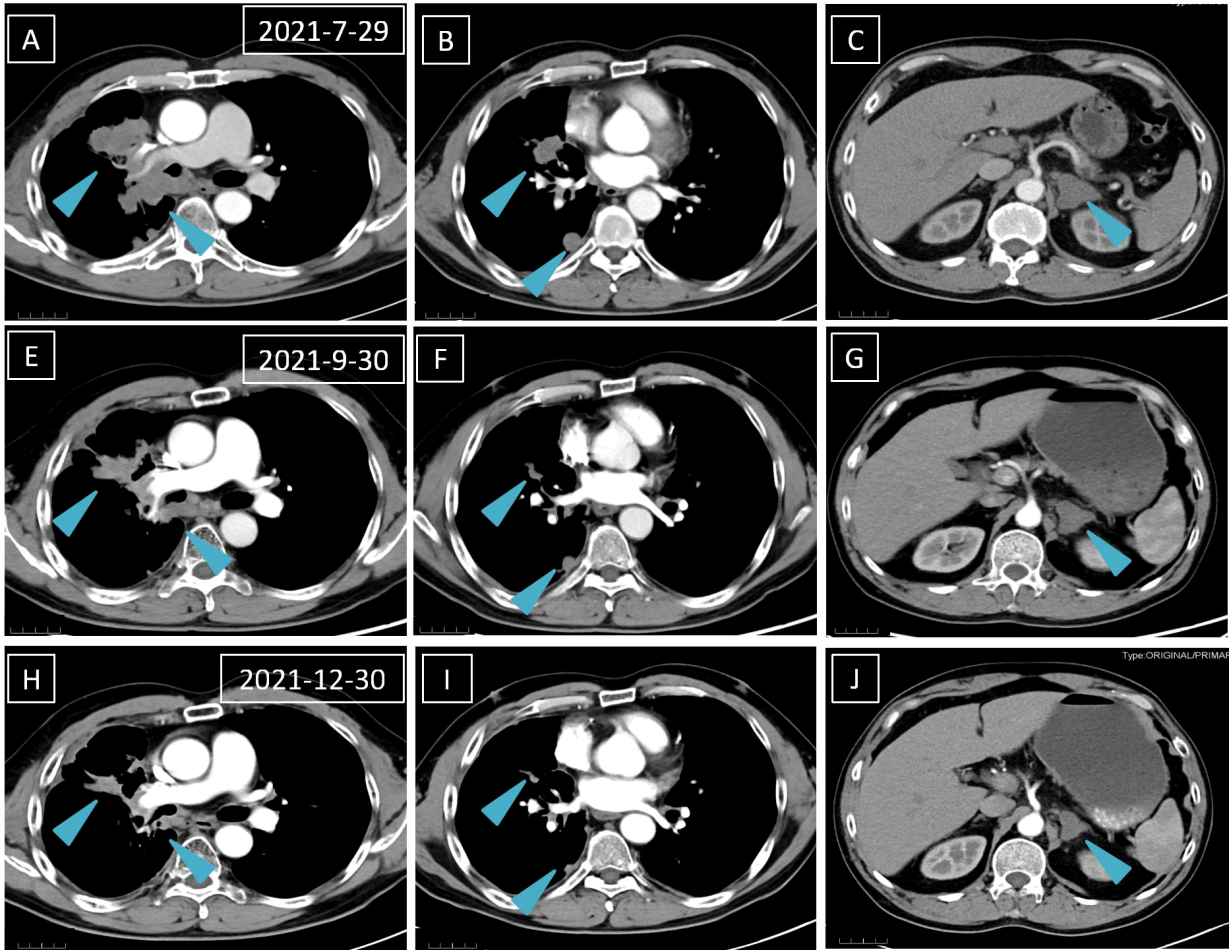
A computed tomography scan is performed before (A–C), 1 month after (D–F), and 4 months after (H–J) trametinib treatment. The blue arrows mean lung and left adrenal gland nodules.
